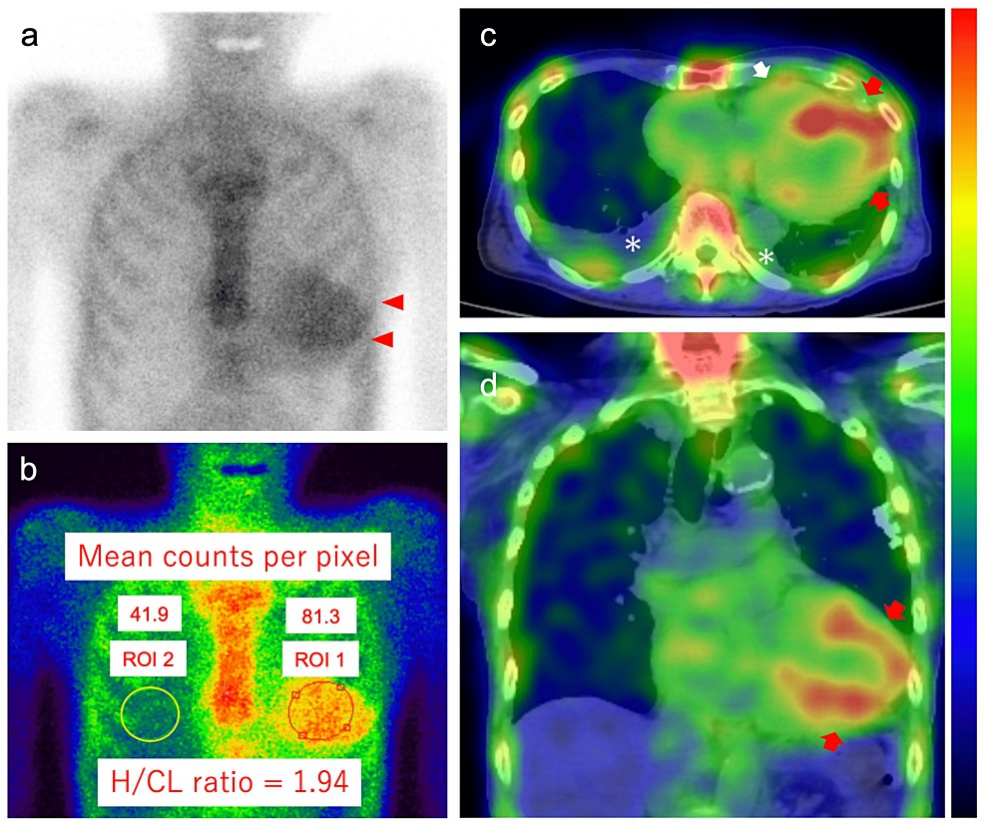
Technetium-99m-pyrophosphate scintigraphy images of Case 1 obtained 2 h after injection of radiotracers Technetium-99m-pyrophosphate planar imaging (anteroposterior view) shows Grade 3 semiquantitative visual scoring of cardiac retention (red arrowheads; high uptake greater than bone): (a) Heart-to-contralateral lung (H/CL) ratio on planar imaging of 1.94, (b) Single-photon emission computed tomographic images with fusion to computed tomographic images show marked tracer uptake in the left (red arrows) and right (white arrow) ventricular myocardium, (c) horizontal plane at the level of the heart, and (d) coronal plane. Regions of interest (ROIs) 1 and 2 indicate the regions of interest positioned to maximize the coverage of the heart without including the adjacent lung and to minimize the overlap with the sternal or focal rib uptake, respectively. Asterisks indicate pleural effusion.
